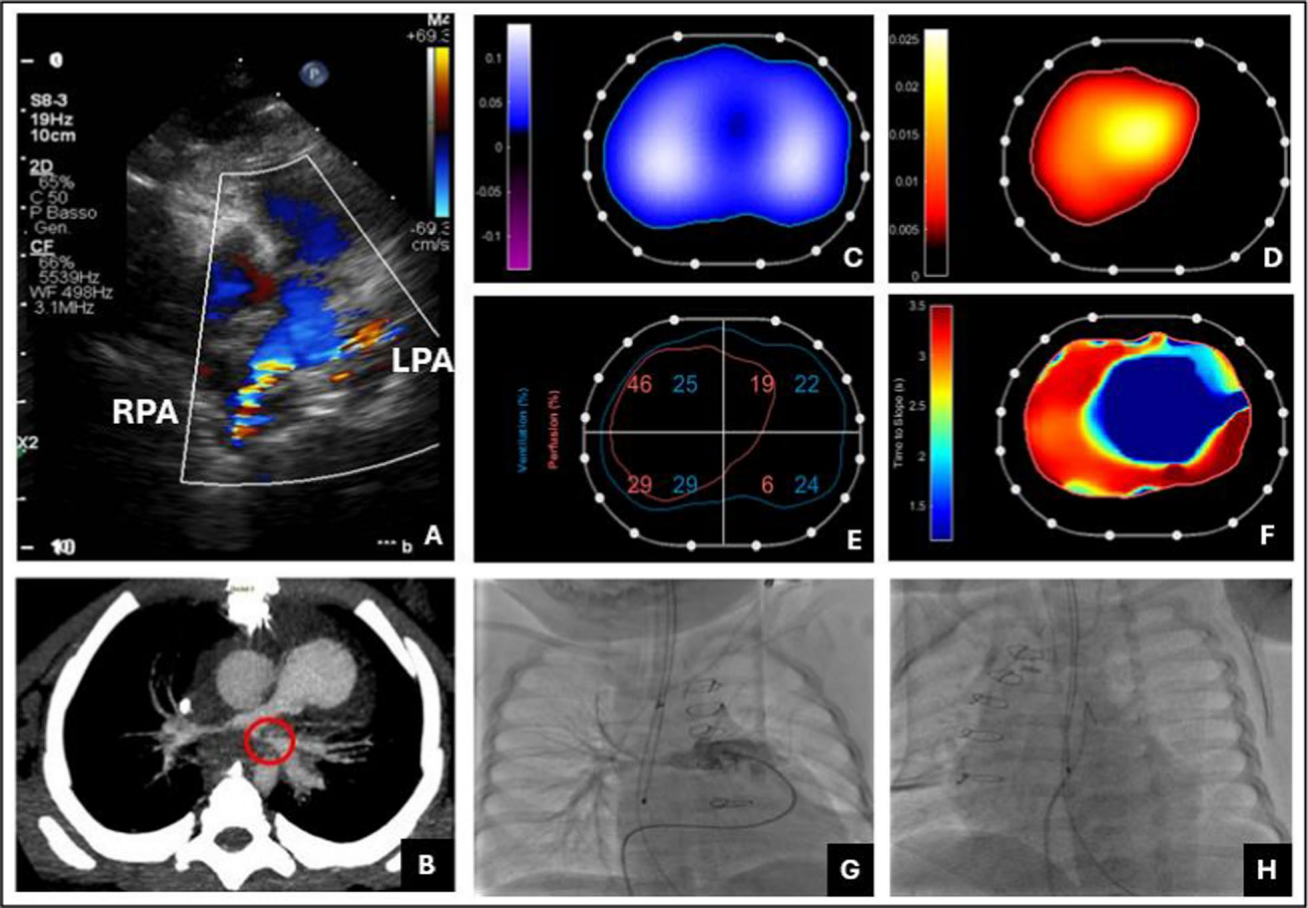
(A) Cardiac ultrasound evaluation with evidence of a diminutive right pulmonary artery (RPA) and marked stenosis of the left pulmonary artery (LPA). (B) CT scan: RPA stenosis and acute angulation of the LPA. Analysis via EIT of global ventilation (C) and perfusion (D) distribution. Pixels are classified as perfused if their perfusion-related impedance decreases of more than 15% of the maximum within the image. (E) Ventilation and perfusion distribution across every ROI (region of interest) with the corresponding matching of the two components: unmatched ventilation 41.6%, unmatched perfusion 0.9%, ventilation–perfusion matching 57.5%. (F) Time to impedance slope in each region: dark blue represents the heart where saline first arrives, followed by yellow and then red areas of the lungs. Cardiac catheterization of the RPA (G) and LPA (H) at the bottom. The patient was ventilated with a PEEP of 5 cmH₂O, a tidal volume of 40 mL, and a respiratory rate of 25 breaths per minute
